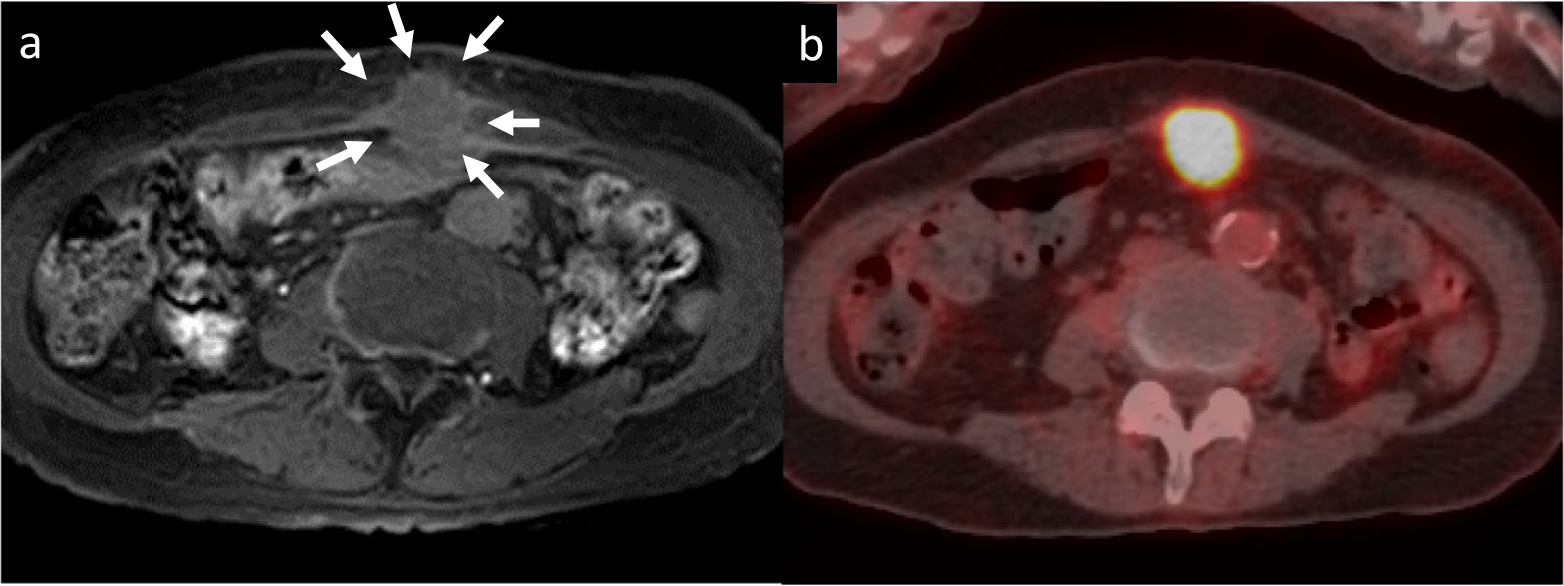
Imaging results 14 months after the initial surgery. a Contrast-enhanced abdominal magnetic resonance imaging showed a 30-mm low-intensity signal from the wound to the transverse colon in T1-weighted imaging that was suggestive of recurrence (white arrows). b
